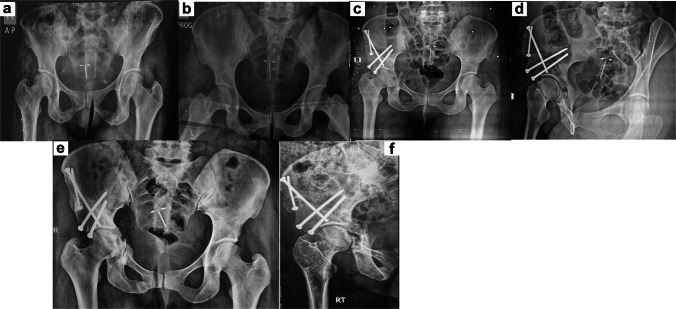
A 29-year-old female with symptomatic adult acetabular dysplasia, predominantly affecting the right hip, treated with PAO. a, b Preoperative radiographs including demonstrating acetabular dysplasia with reduced LCEA and increased AI. c, d Immediate postoperative radiographs showing adequate acetabular reorientation with correction of both LCEA and AI, stabilized using four 4.5-mm cortical screws. e, f Final follow-up radiographs at 8 years demonstrating maintained correction, complete osteotomy union, stable fixation, and absence of secondary complications
